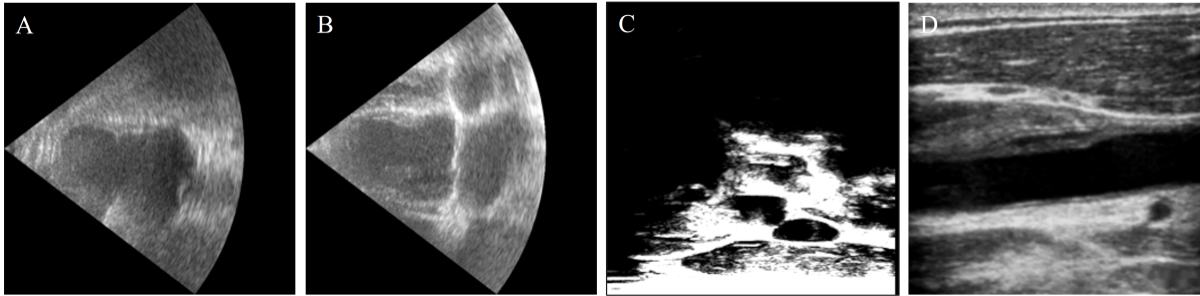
Cardiac ultrasound (US) and carotid US. (A) Cardiac US (2 heart chambers). (B) Cardiac US (4 heart chambers). (C) 3D carotid US. (D) 2D carotid US.
